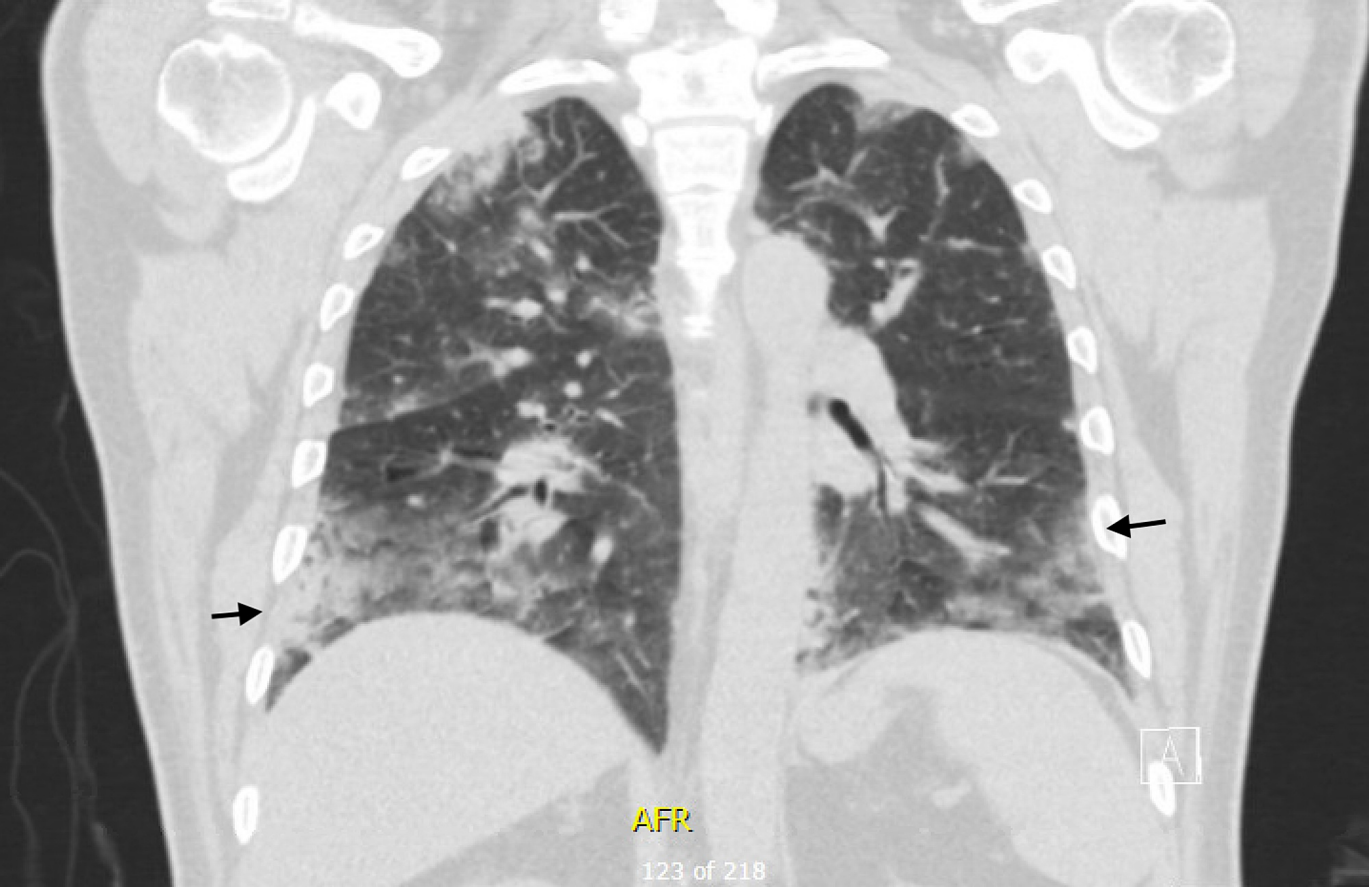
Coronal view of CT chest with diffuse peripheral ground glass, consolidative, nodular opacities in all lobes consistent with multifocal pneumonia (black arrows). CT, computed tomography
